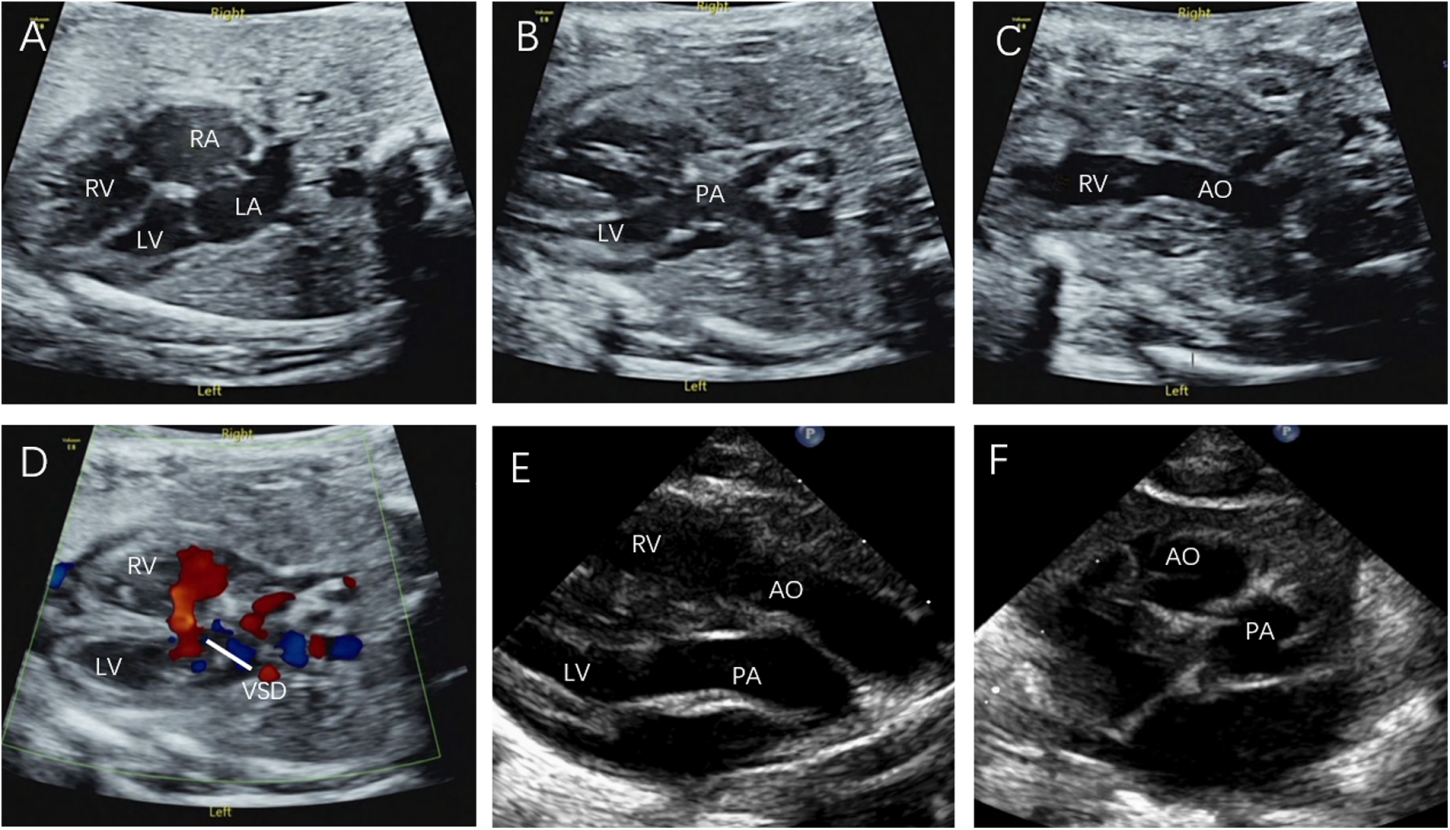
Prenatal and postnatal diagnosis of D-TGA. (A–D) D-TGA with VSD at 24 w and 6 d gestation. (E) Parasternal long-axis view: The two great arteries are aligned in parallel, with the aorta located anteriorly on the right, originating from the right ventricle, and the pulmonary artery located posteriorly on the left, originating from the left ventricle. (F) The short axis view of the great arteries: The aorta and pulmonary artery are circumferential and adjacent rather than travelling normally (the longitudinal pulmonary artery surrounds the circular aorta).
